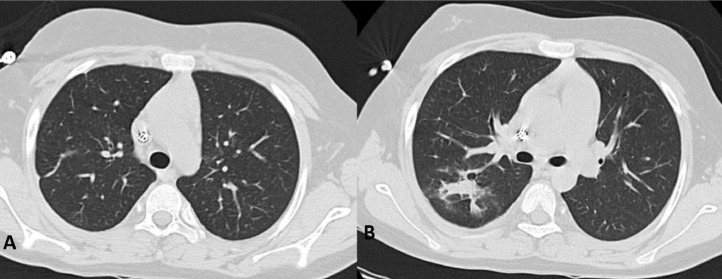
Axial (A, B) lung view of a follow-up thoracic CT scan without contrast: The previously visible cavitary lung lesions had disappeared.
